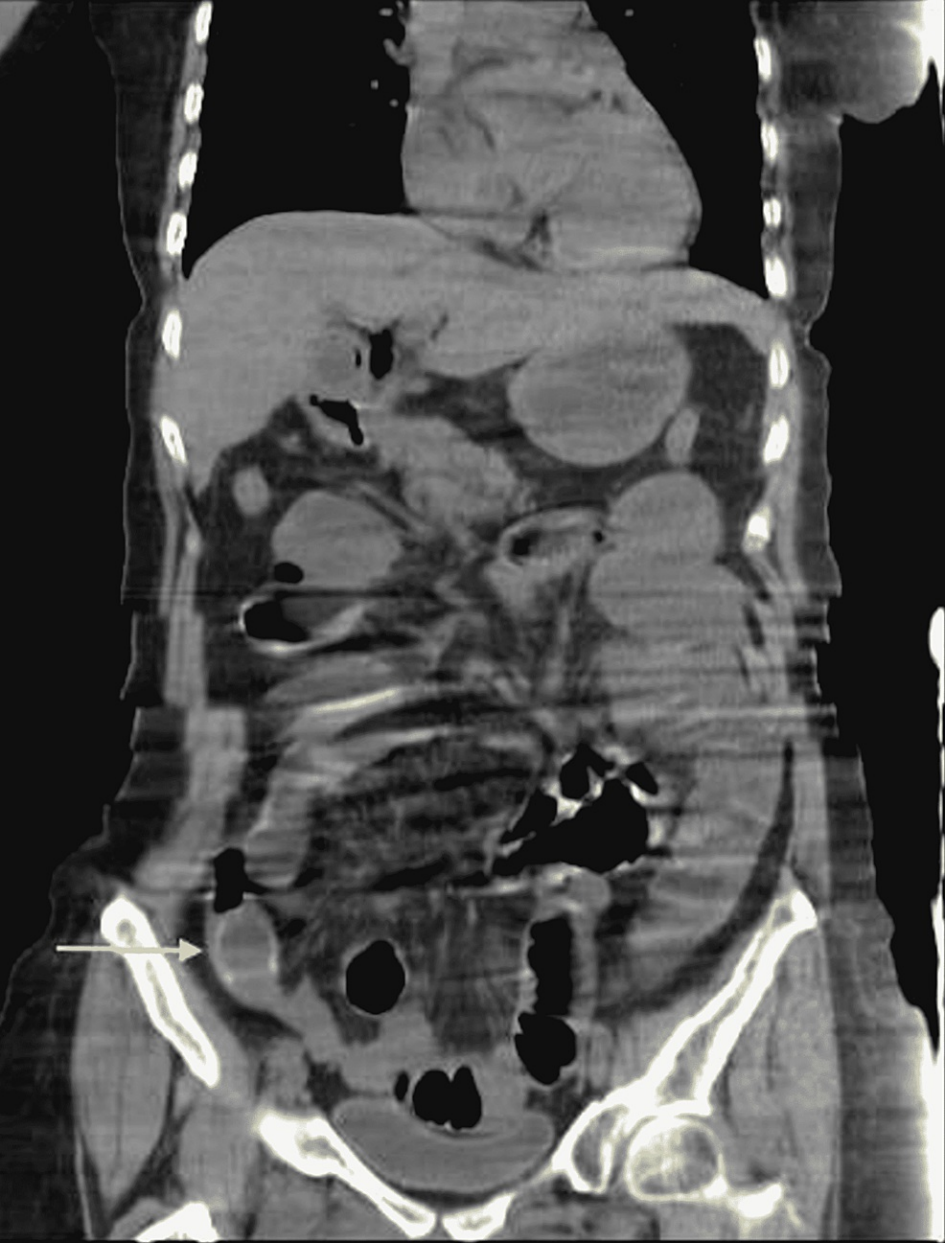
Coronal view of abdominal computed tomography Gallstone in the distal ileum.
